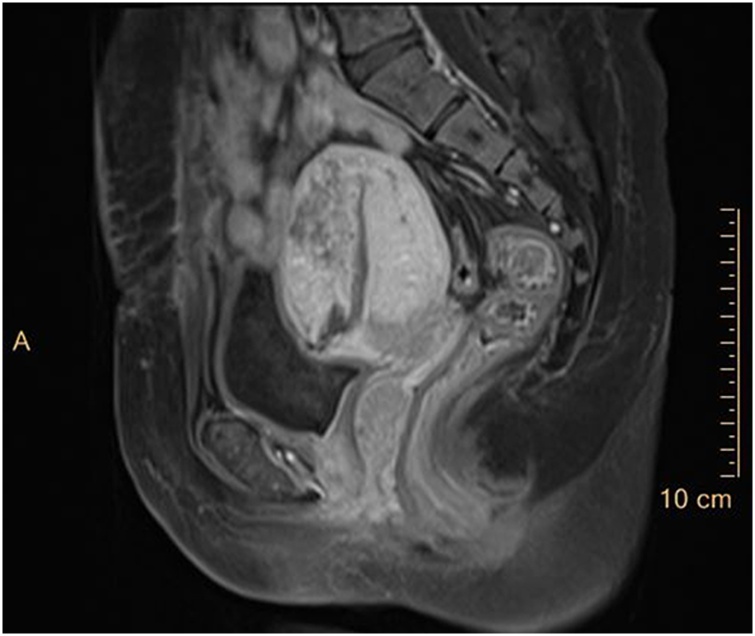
MRI Sagittal (white arrow refers to cancer tissue).
